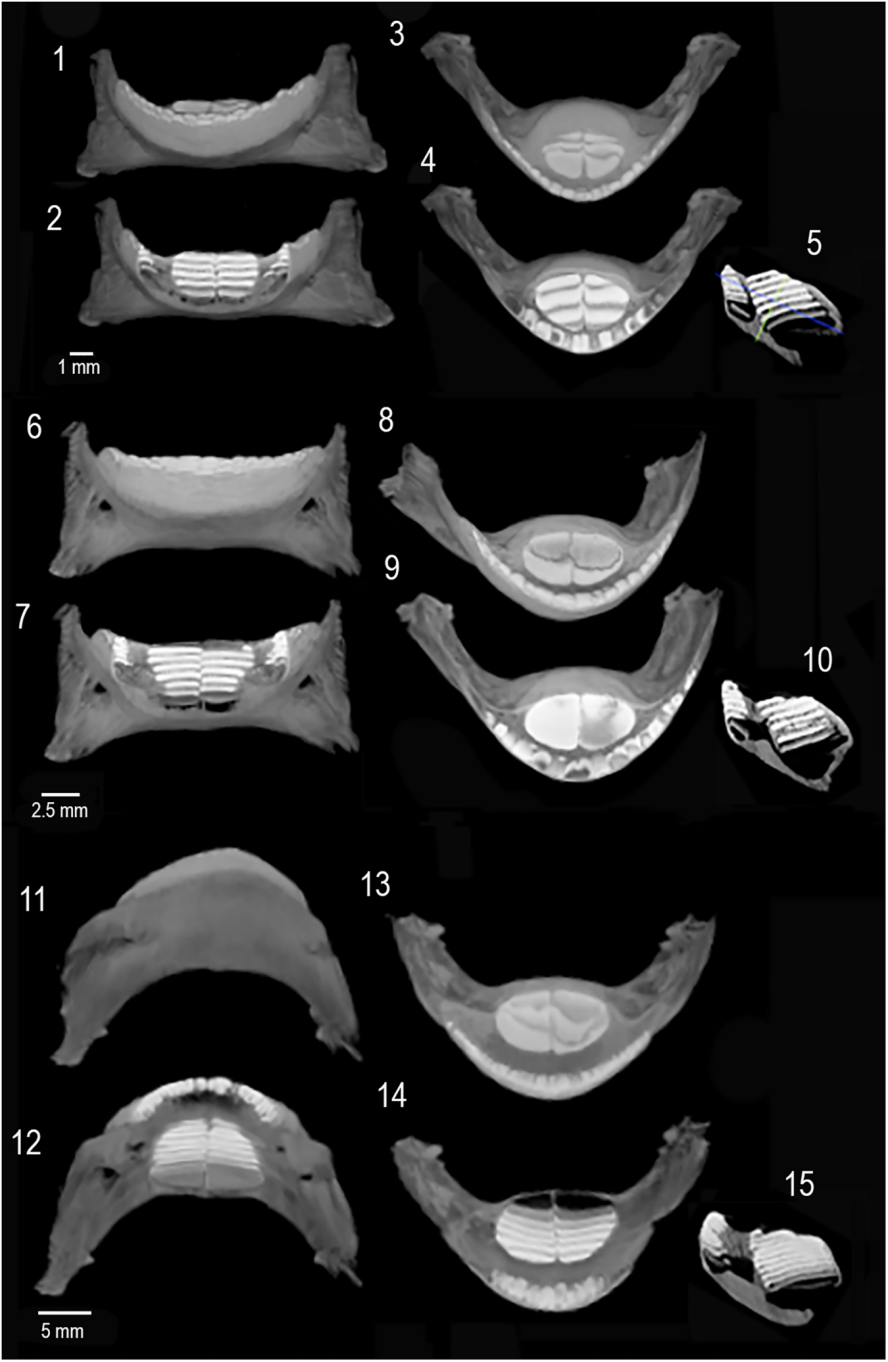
Micro CT plates of ontogenetic series of jaws from extant *Chilomycterus spinosus* from Santos, São Paulo, Brazil. 1–5, UFF ZO314: 92 mm total body length (TL), 72 mm standard length (SL); 6–10,UFF ZO312: 159 mm TL, 120 mm SL; 11–15, UFF ZO315: 276 mm TL, 230 mm SL.
